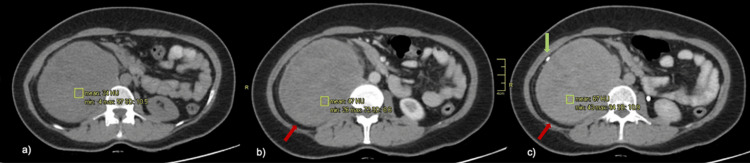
Contrast-enhanced abdominal CT characteristics of the mass The mass shows well-circumscribed borders with soft-tissue density and progressive post-contrast enhancement. Phases: (a) non-contrast phase (34 HU). (b) Arterial phase (47 HU), with the mass indicated by a red arrow. (c) Delayed phase (67 HU), showing the mass (red arrow) and right ureteral displacement caused by the lesion (green arrow). CT: computed tomography
